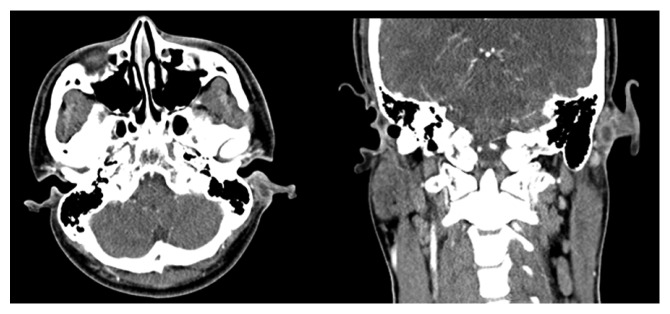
A CT scan with contrast shows a left rim-enhancing cyst in the postauricular crease.
